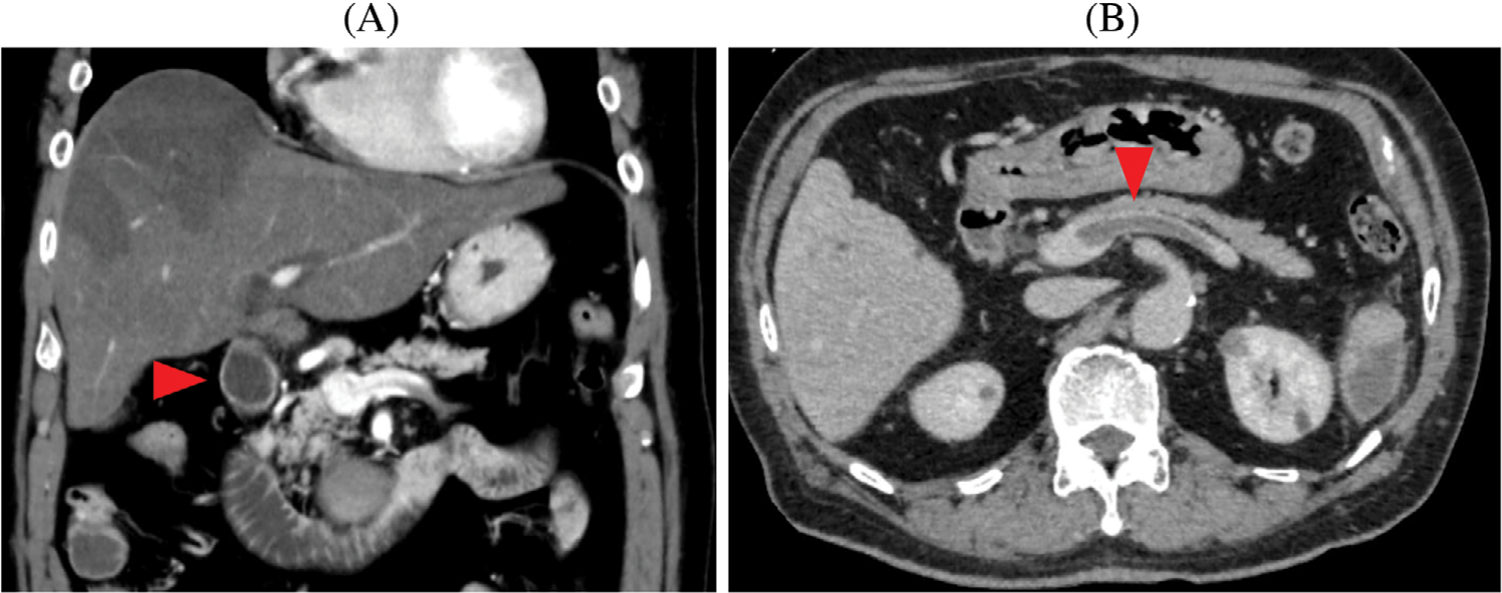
Coronal plane (A) and transverse plane (B) of an enhanced CT of the trunk. Arrowheads indicate thrombosis in the inferior vena cava (A) and the splenic artery (B)
